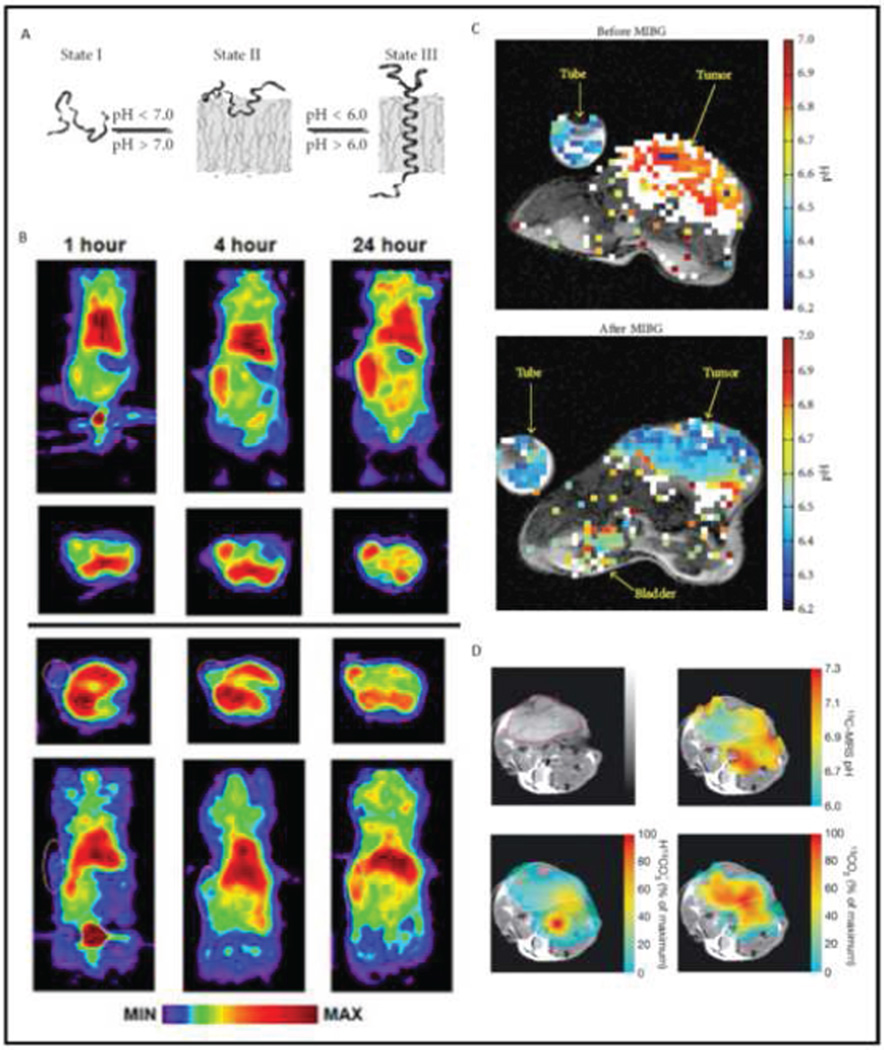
Tumor acidosis can be interrogated with noninvasive imaging. A) The pH low-insertion peptide (pHLIP) inserts itself in the membranes of cells in low pH microenvironments. B) Coupled with a 64Cu contrast agent, the pHLIP protein can be used to measure tumor pHe with positron emission topography. C) Using an FDA approved contrast agent, acidoCEST MRI measures tumor extracellular pH before and after drug treatment. D) Hyperpolarized 13C MRI can be used to measure tumor pHe by measuring the ratios of hyperpolarized bicarbonate to hyperpolarized carbon dioxide. Reproduced with permission from references 96, 99, 102, and 103.
